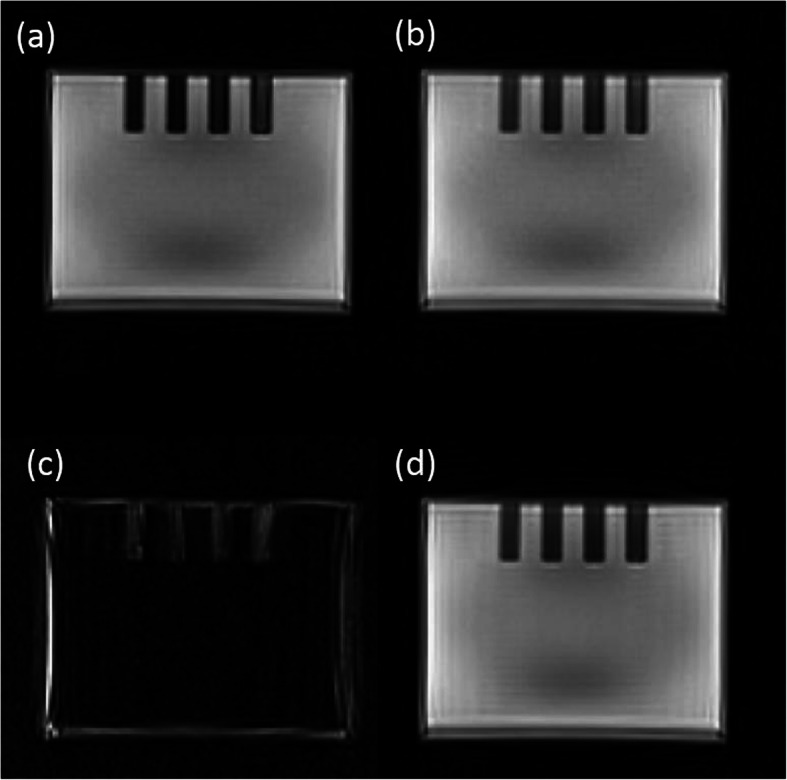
Dixon MR images from the standard Dixon-based AC approach in the Biograph mMR PET/MR system. a in-phase image, b opposed-phase image, c “fat” image, d water image
